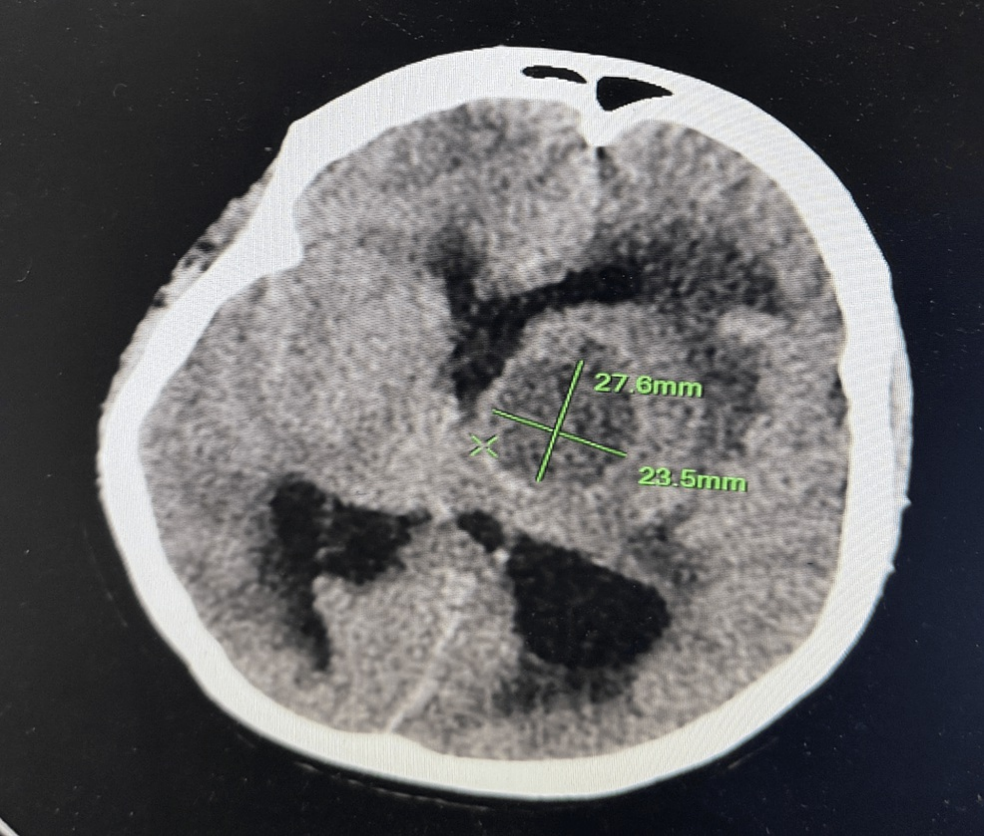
Axial NCCT scan showing AP and TV dimensions of the lesion measuring 27.6×23.5 mm, respectively. The dimensions of the abscess are marked with green lines: 27.6 mm in the AP direction and 23.5 mm in the TV direction. NCCT, non-contrast-enhanced computed tomography
